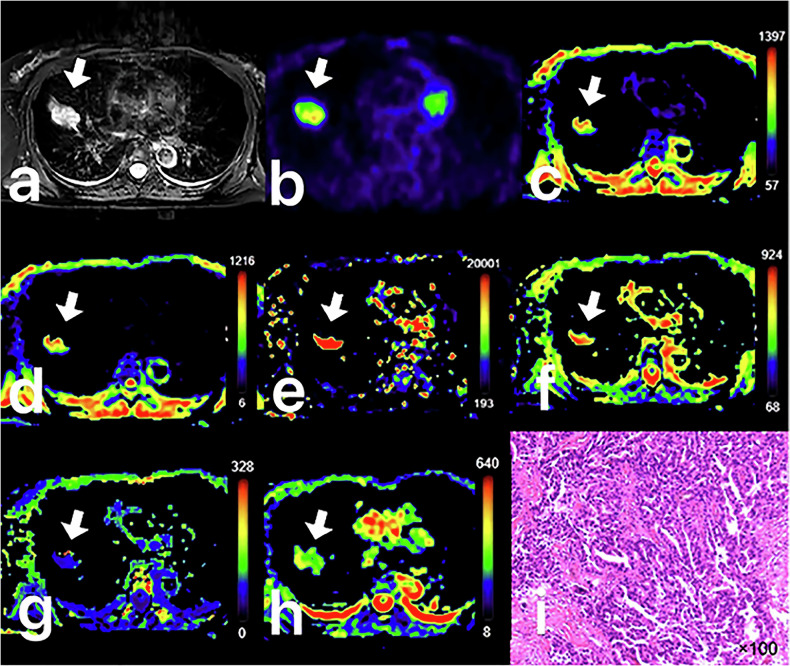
Female, 51 years old, with adenocarcinoma (AC) in the right upper lobe, maximum diameter is 3.1 cm. a Image of axial T2WI with fat saturation pre-pulse. b Fusion image of the SUV map and the ultrashort echo time map. c Pseudo colored map of ADCstand. d Pseudo colored map of D. e Pseudo colored map of D*. f Pseudo colored map of f. g Pseudo colored map of MK. h Pseudo colored map of MD. i Pathological image that displays negative lymphovascular invasion
